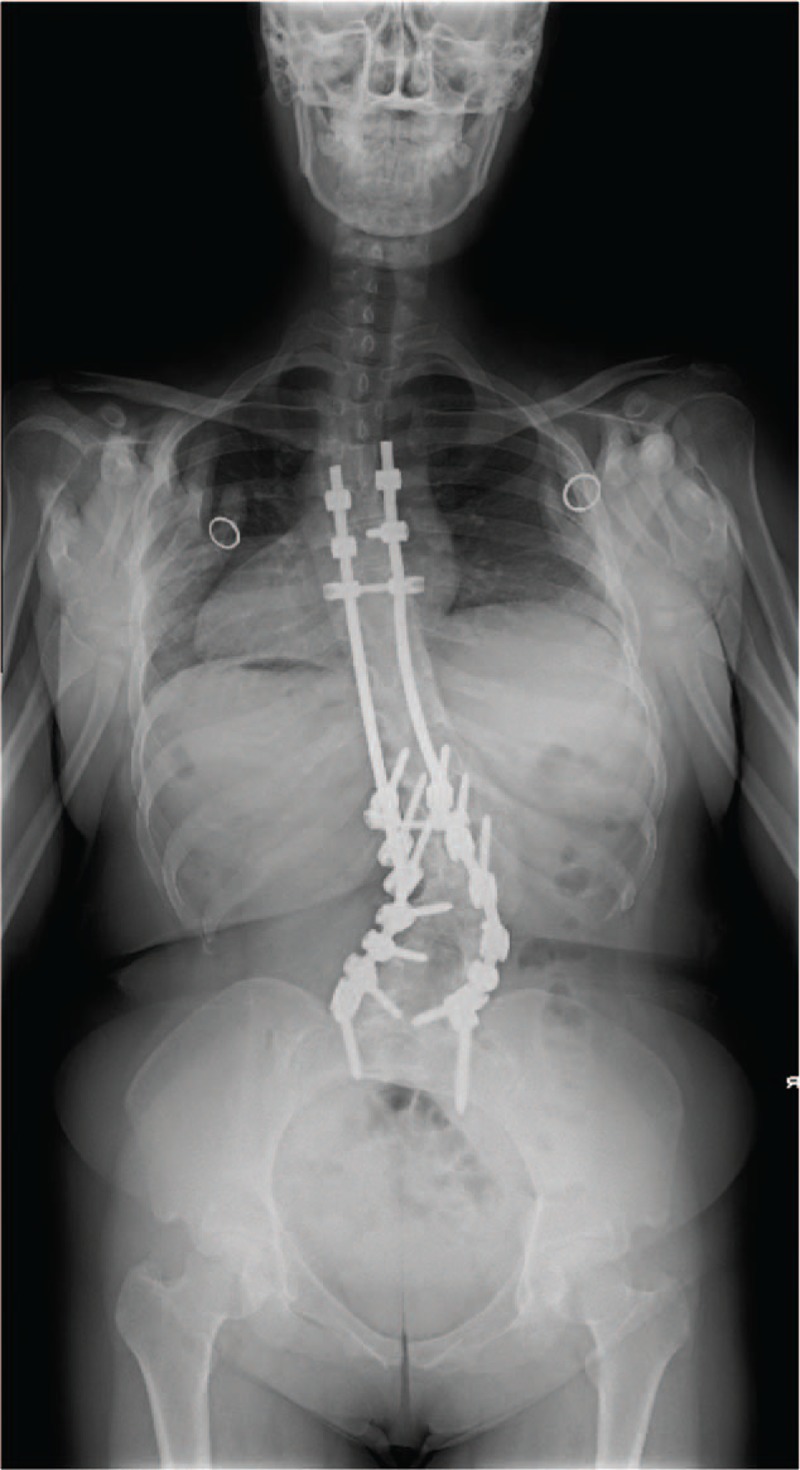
Postoperative anteroposterior radiograph showing the scoliotic lower curve corrected to 31° and upper curve corrected to 19°.
